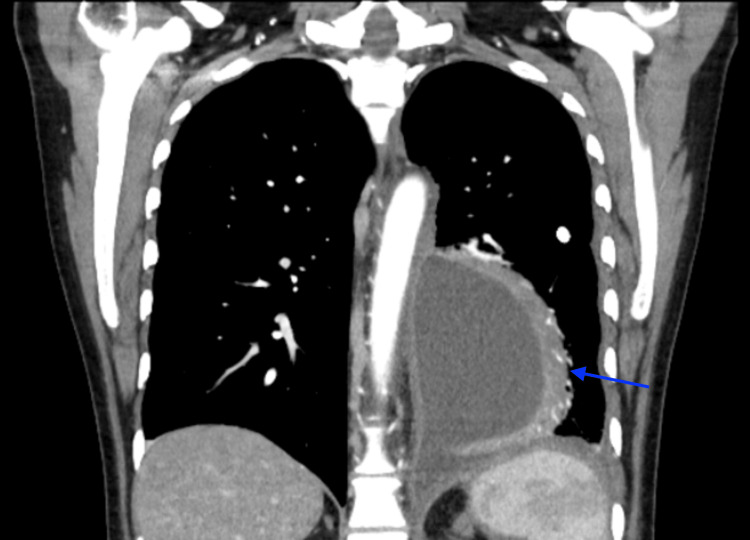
CT in coronal view, blue arrow pointing to rim-enhancing left lower lobe pulmonary abscess/empyema
